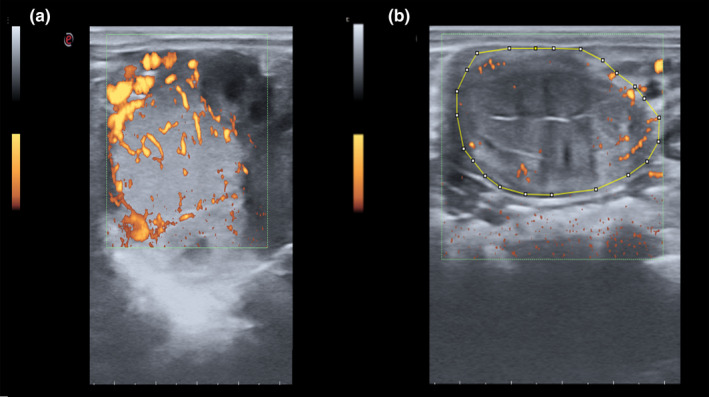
(a) Power Doppler ultrasound of a functional CL of 8 days postovulation in a mare. The day 8 postovulation is the day with the highest blood supply to the CL and with the highest production of progesterone. Power Doppler ultrasound is the most sensible technique to assess the vascularization of CL. (b) Endometrial blood flow evaluated with power Doppler ultrasound. Computer‐assisted pixel analysis using Image J software has been performed in research work in order to semi‐quantitatively evaluate the vascularization and echotexture of different organs based on the area and intensity of pixels identified in the image
